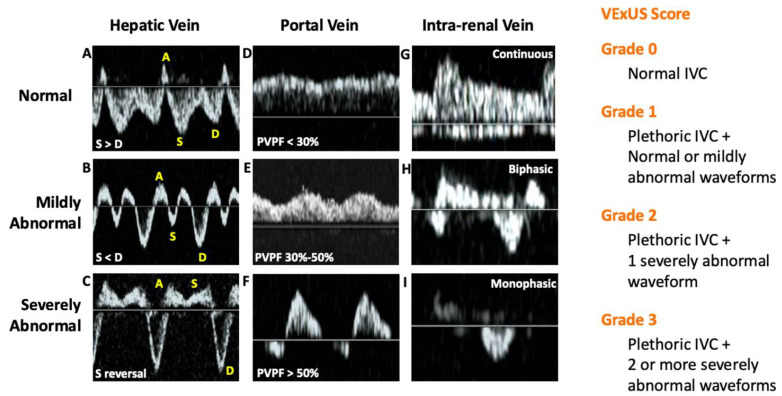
Left: Representative waveforms of normal and abnormal hepatic (HVD), portal (PVD), and intra-renal venous Doppler (IRVD). (A) Normal HVD, note S wave > D wave; (B) moderately abnormal HVD, note S wave < D wave; (C) severely abnormal HVD, note reversed S wave; (D) normal PVD, pulsatility fraction < 30%; (E) moderately abnormal PVD, pulsatility fraction between 30 and 50%; (F) severely abnormal PVD, pulsatility fraction >50%; (G) normal IRVD, continuous pattern; (H) moderately abnormal IRVD, biphasic pattern, and (I) severely abnormal IRVD, monophasic pattern. Right: VExUS grading system.
